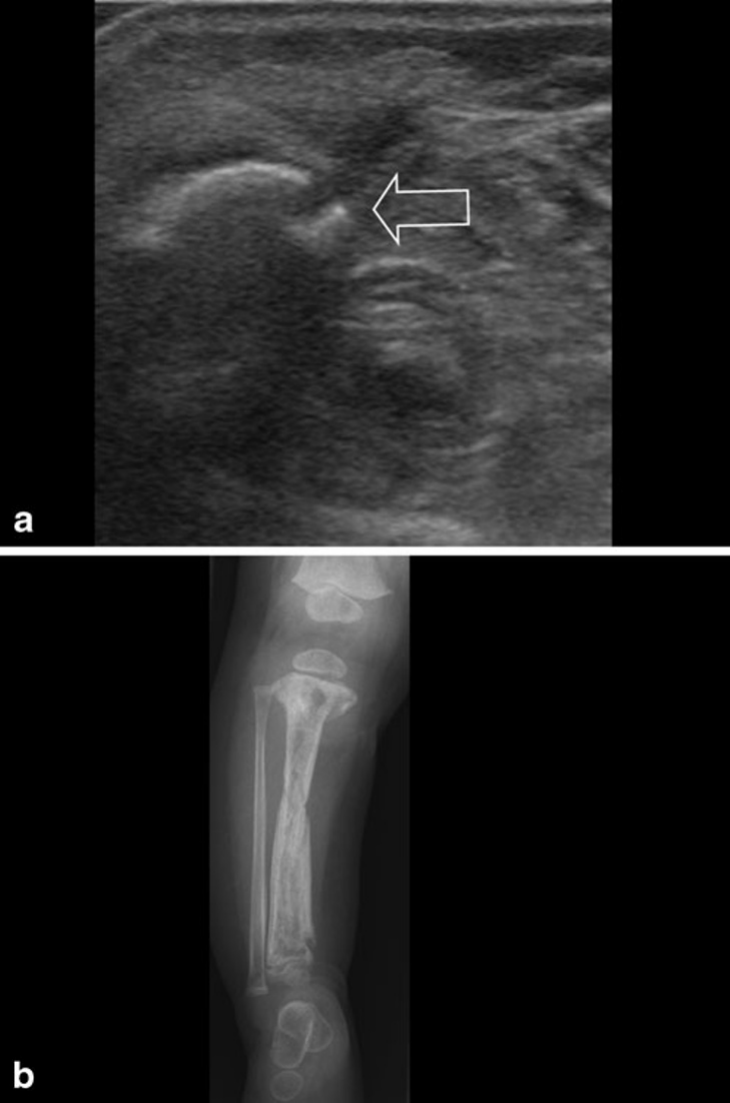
a Same patient as in Fig. 4 was treated with antibiotics for 1 month. Because of low-grade fever, ultrasonography was repeated. A rest of the abscess was seen as well as extensive cortical destruction at multiple locations (*arrow* shows one). b Conventional image was performed to evaluate the extensiveness, showing complete involvement of the tibia, with fracture lines in the proximal and distal metaphysis, and mid diaphysis
